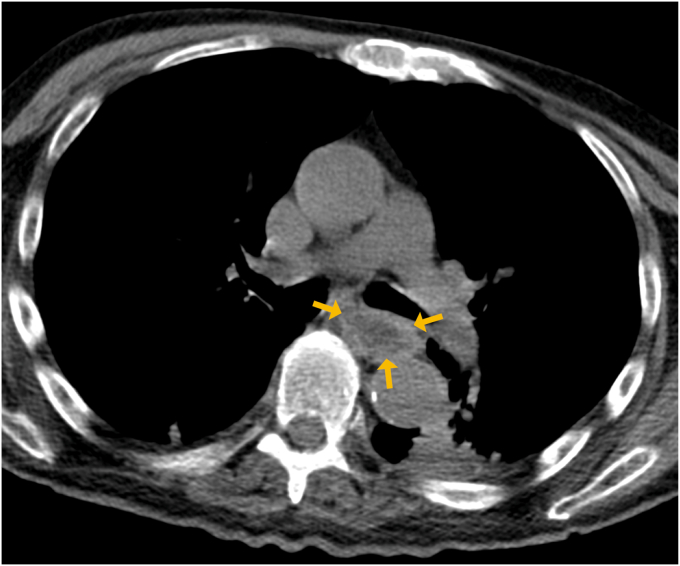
Axial contrast-enhanced CT scan showing a well-circumscribed hypodense intraluminal esophageal lesion (*yellow arrows*), with no evidence of wall invasion or involvement of adjacent structures.
